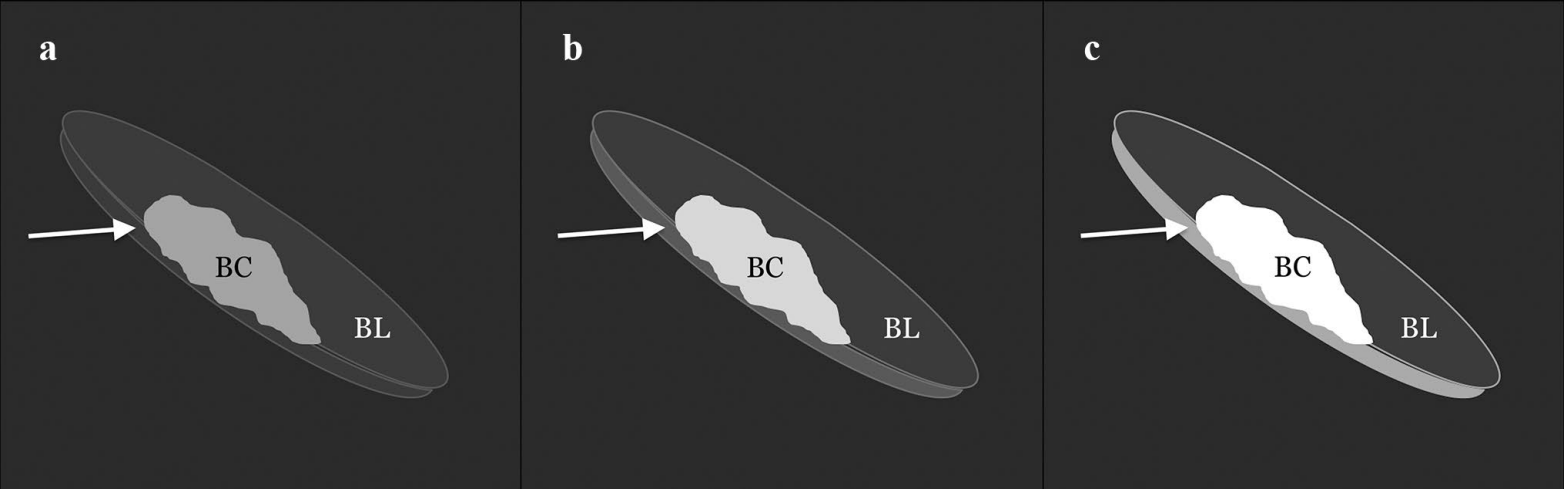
Three-point scale used to assess tumor conspicuity on DWI: a hyperintense lesion, but only slightly demarcated from the background and/or poor tumor–wall interface, b hyperintense lesion, well demarcated from the background with good tumor–wall interface, and c hyperintense lesion with excellent background suppression and optimal tumor–wall interface. BC = bladder cancer; arrow = bladder wall; BL = bladder lumen
